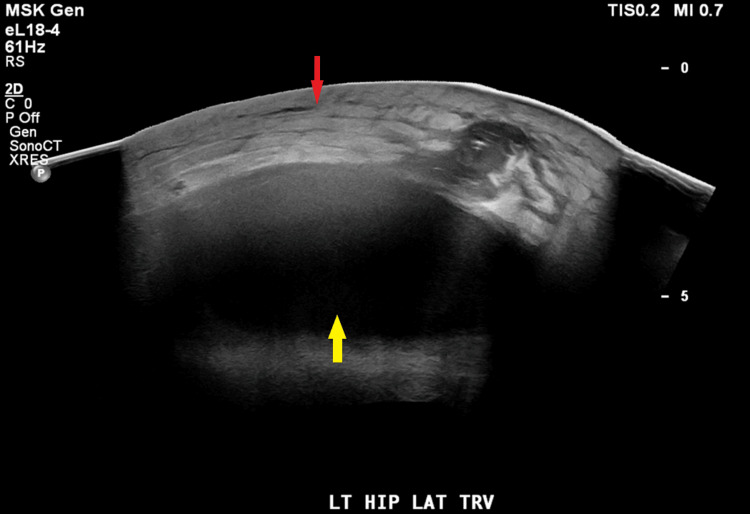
Ultrasound of left lower extremity taken during April hospitalization Ultrasound showed a large fluid collection (yellow) overlying the left hip/thigh with overlying soft tissue edema (red).
